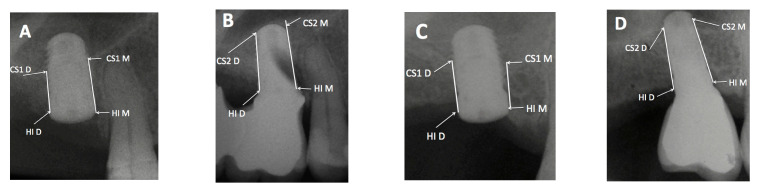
(A): Post-surgical periapical X-ray of Patient 1. (B): Periapical X-ray at 18 months of Patient 1. (C): Post-surgical periapical X-ray of patient 2. (D): Periapical X-ray at 18 months of patient 2. In all cases, bone gain was obtained between baseline (A,C) and the 18-month follow-up (B,D) can be seen.
